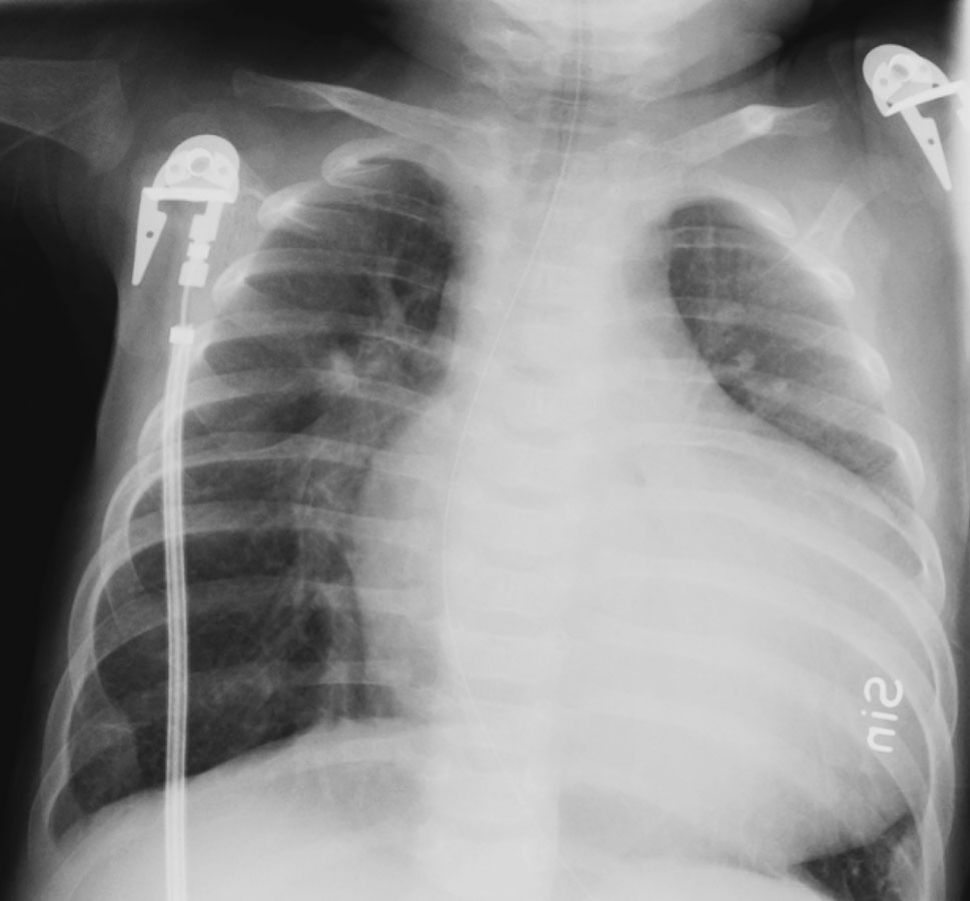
The chest X-ray at the time of presentation in the 4 month old proband, showing the severely enlarged heart
